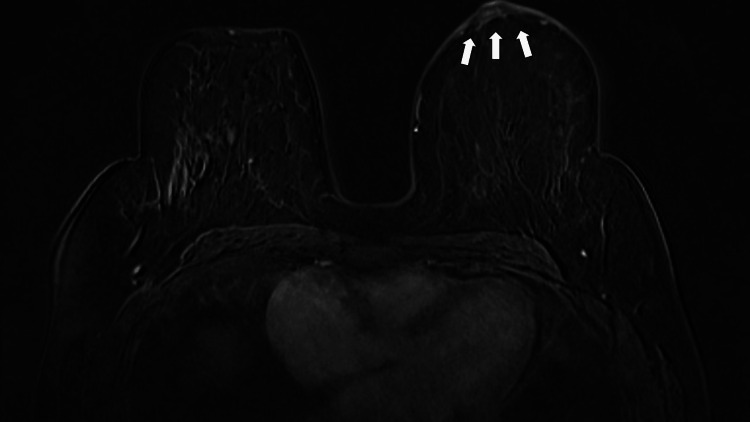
Breast magnetic resonance imaging. The digital subtraction T1-weighted contrast-enhanced MRI shows slight uptake in the left subareolar area (arrows).
